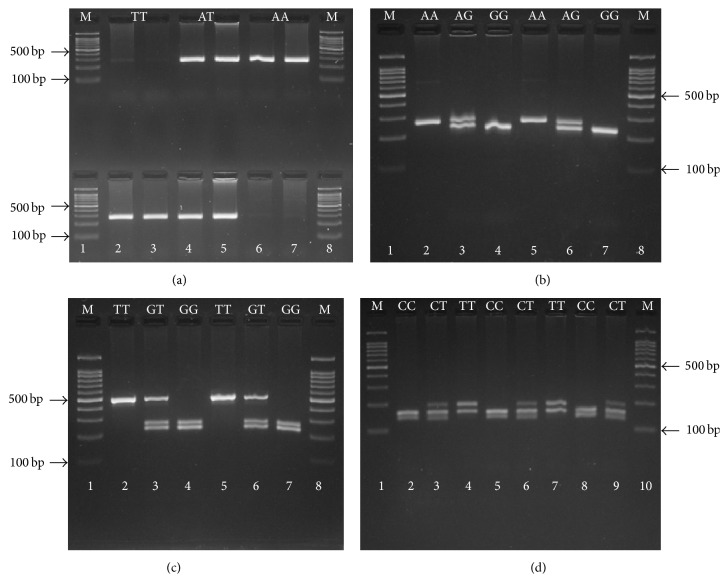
Electrophoresis pattern of ARMS-PCR for detection of IFN-*γ* (+874A/T) (a) and RFLP-PCR IFN-*γ*R1 (-611A/G), (+189T/G), and (+95C/T) gene polymorphism (resp., (b), (c), and (d)). (a) Lines 1 and 8: marker 100 bp; Lines 2 and 3: product sizes were 264 bp for forward T allele primer (FT) and reverse primer (R); Homozygote TT; Lines 6 and 7: product sizes were 264 bp for forward A allele primer (FA) and reverse primer (R); Homozygote AA; Lines 4 and 5: product sizes were 264 bp for FT, FA, and R; Heterozygote AT; (b) Lines 1 and 8: marker 100 bp; Lines 2 and 5: Homozygote AA; Lines 4 and 7: Homozygote GG; Lines 3 and 6: Heterozygote AG; (c) Lines 1 and 8: marker 100 bp; Lines 2 and 5: Homozygote TT; Lines 4 and 7: Homozygote GG; Lines 3 and 6: Heterozygote GT; (d) Lines 1 and 10: Marker 100 bp, Lines 2, 5, and 8: Homozygote CC; Lines 4 and 7: Homozygote TT; Lines 3, 6, and 9: Heterozygote CT.
